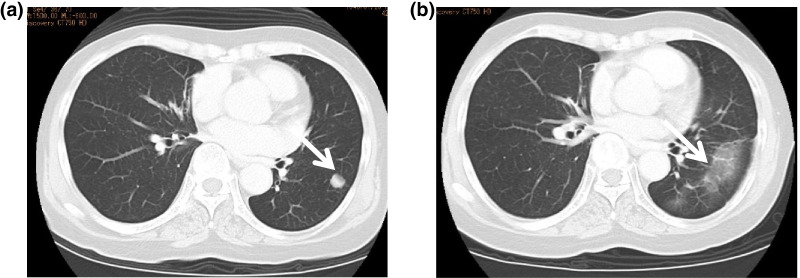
a Chest CT scan showing the presence of lung metastases (white arrow). CT, computed tomography. b Chest CT scan showing the disappearance of lung metastases after radiotherapy (white arrow). *CT* computed tomography
